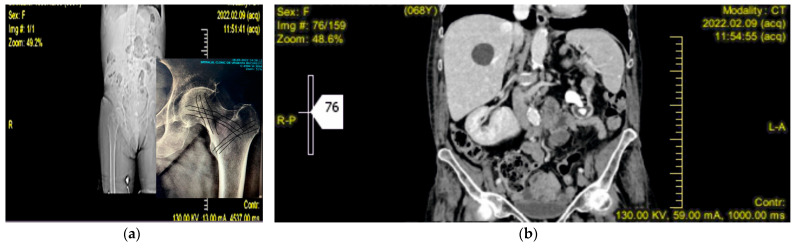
Case 1. (a) X-ray radiograph with inset showing the trabeculae of the femoral neck; (b) CT before fracture.
